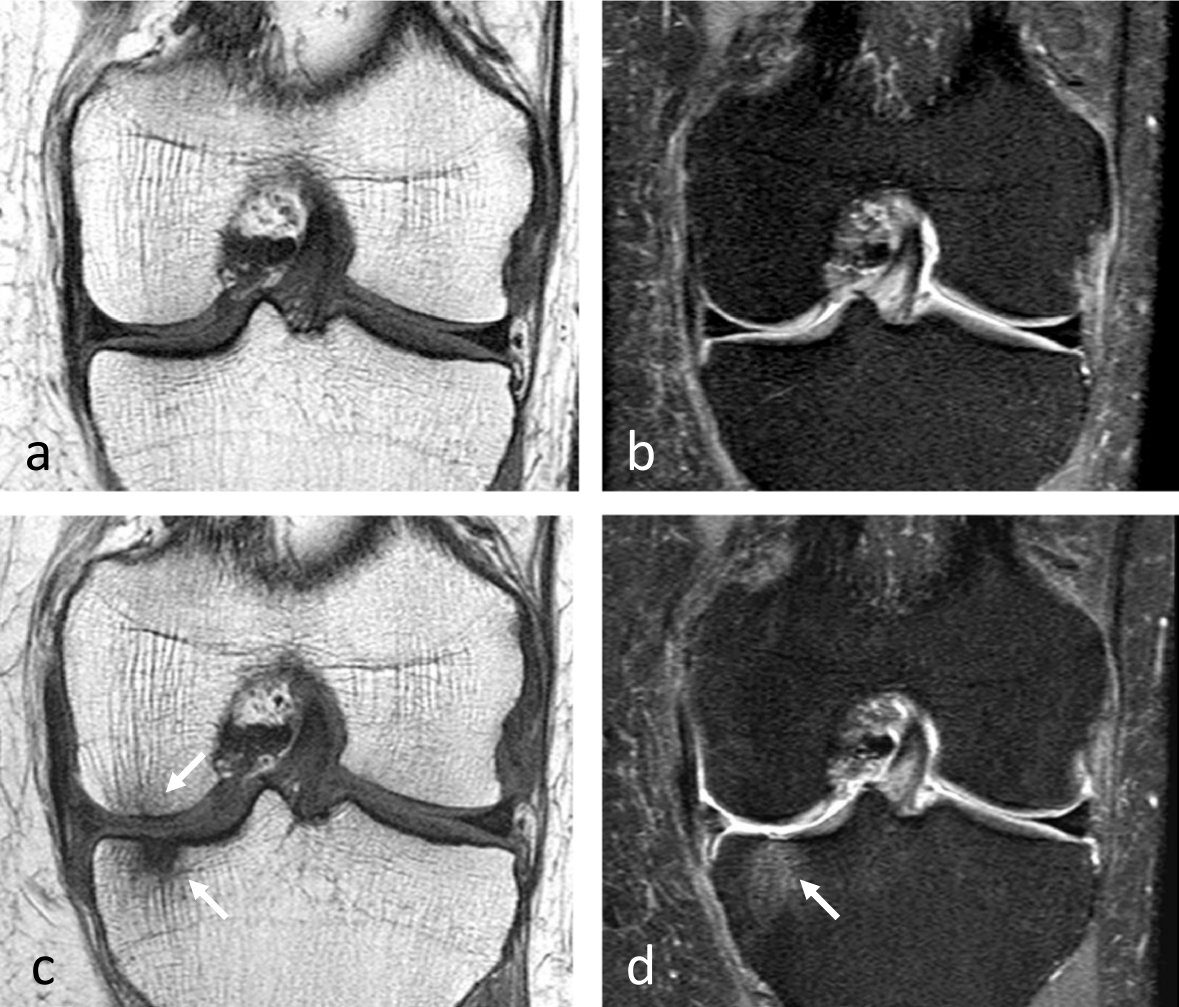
Consequence of a meniscectomy on a subchondral bone. a, b In this middle-aged subject, the coronal T1-weighted (a) and fat-suppressed T2-weighted (b) MR images show no abnormality. c, d Six months after resection of the (normal!) medial meniscus, T1-weighted (c) and fat-suppressed T2-weighted (d) MR images show a BME-like pattern in the lower pole of medial condyle and on the medial tibial plateau (arrows in c and d)
